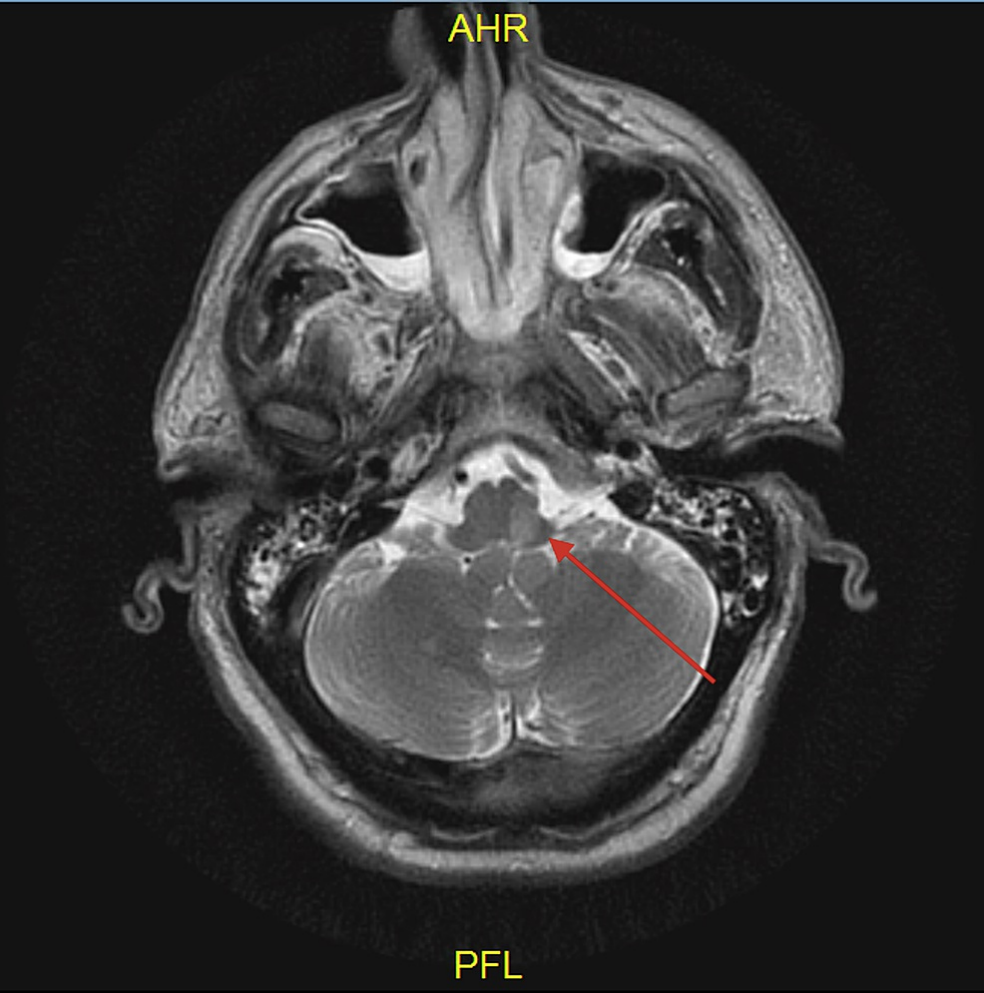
MRI head showing left medullary infarction on axial view. MRI: magnetic resonance imaging.
